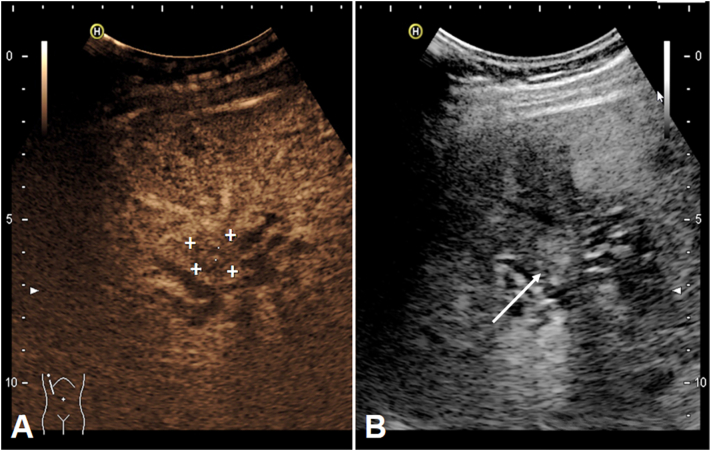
(A) CEUS showing a central lesion of 1.7 cm in diameter (marked with crosses) with weak venous washout between liver segments V and VIII. (B) Conventional sonographic imaging showing the lesion (arrow) and dilated bile ducts distal of the lesion.
